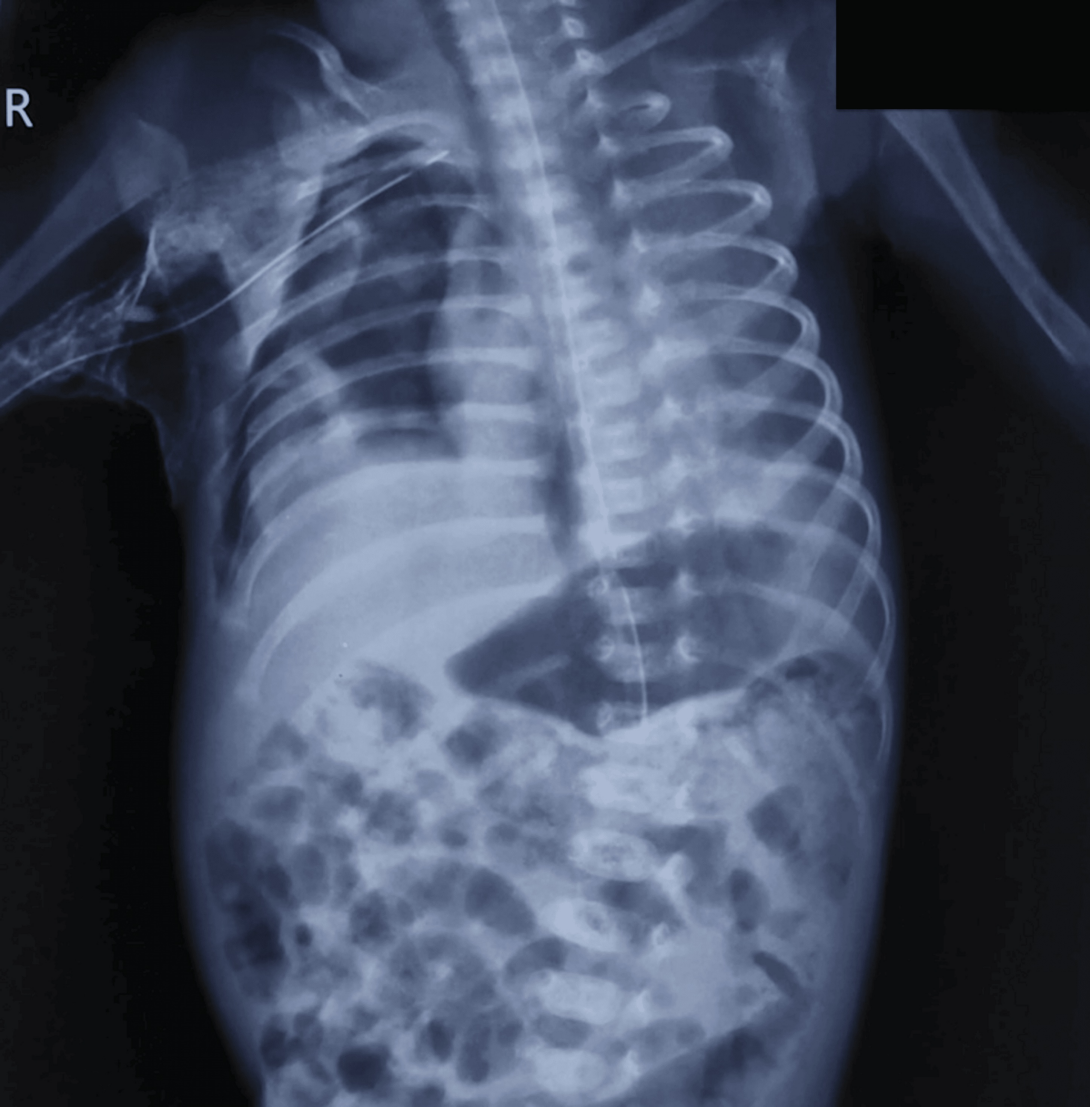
Chest X-ray on day 25 showing a persistent right-sided pneumothorax, evidenced by increased radiolucency in the right hemithorax with visible lung edge and partially collapsed right lung. Note the absence of vascular markings laterally, indicating the presence of free air in the pleural space.
